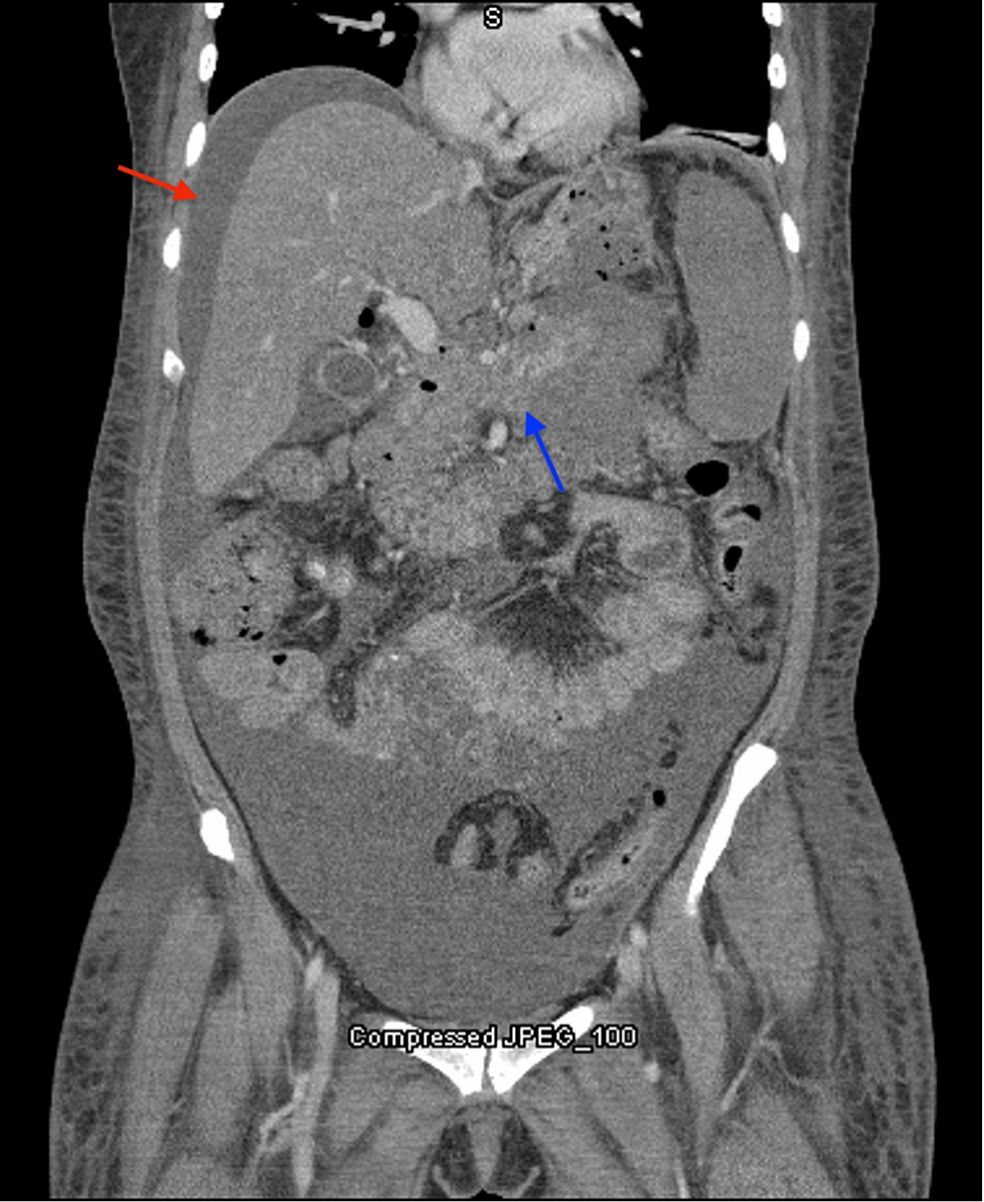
The CT scan shows ascites (red arrow) and the peripancreatic fluid collection (blue arrow).
